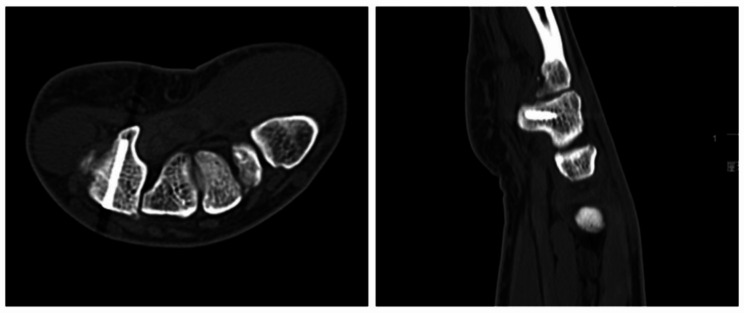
The CT images showed that the hamate hook fracture had healed at three months
